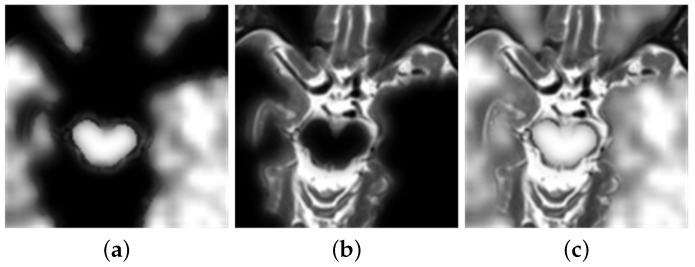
Image fusion. (a) The product of MRI and final decision map. (b) The product of PET and complementary set of final decision map. (c) Final fusion image.
